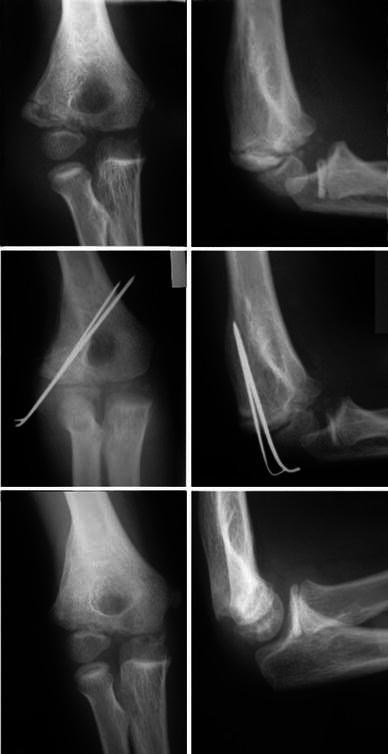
9-year-old male, fracture healed at 5 weeks, X-ray at 1 year demonstrates healing with lateral hypertrophy
